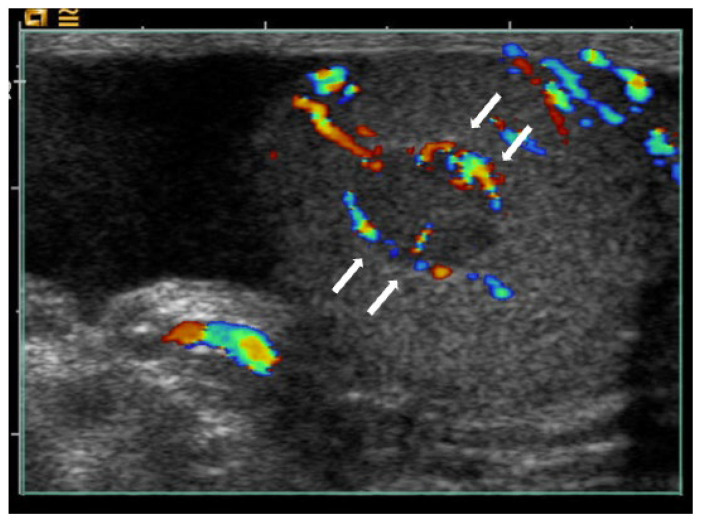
Peripheral vascularity in a small benign Leydig cell tumour. Longitudinal colour Doppler ultrasound images of the left testis show a well-circumscribed hypoechoic intratesticular lesion measuring 5 mm, demonstrating peripheral vascularity (short arrows).
